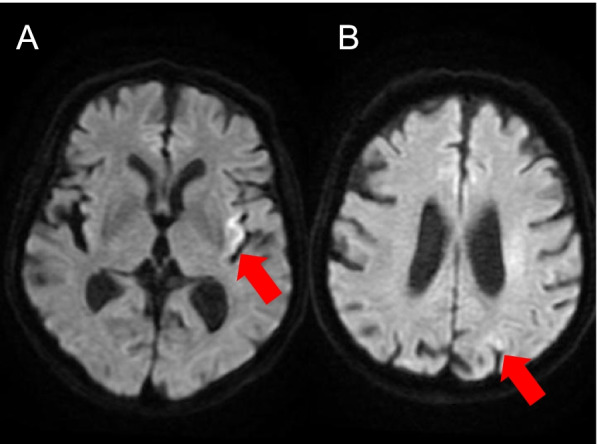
Day 0 DWI. A and B Initial acute ischemic stroke (red arrows) on DWI sequence at day 0. DWI indicates diffusion-weighted imaging
